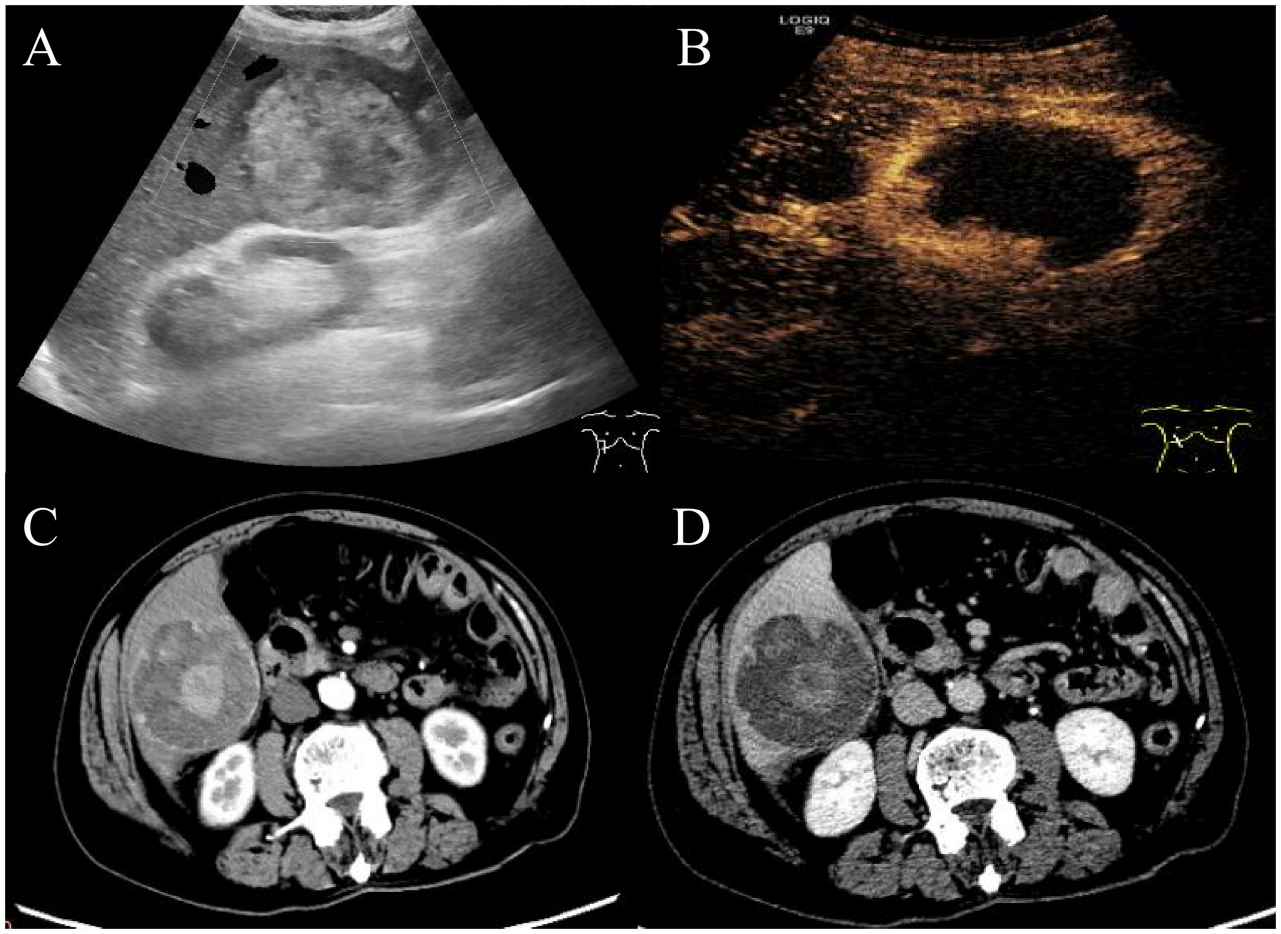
Abdominal ultrasound and enhanced CT imaging of the manifestations of liver lesions. Abdominal ultrasound and contrast-enhanced ultrasound showed a well-defined hyperechoic lesion in the right posterior hepatic lobe (A, B). Contrast-enhanced CT revealed a hyperechoic tumor in hepatic segment VI with peripheral hyperdensity and central hypodensity with heterogeneous hyperdensity during the portal phase (C, D).
